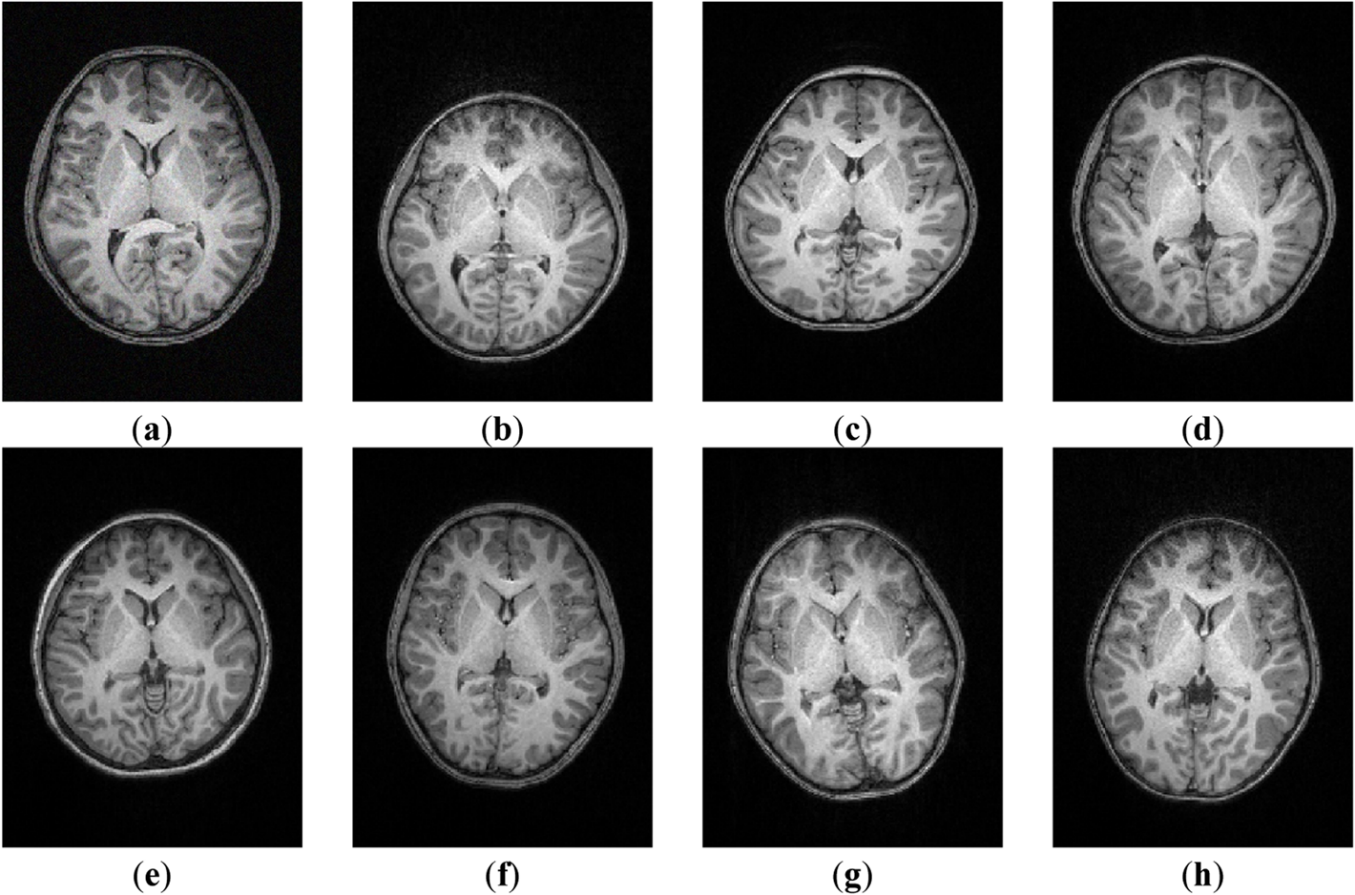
Real human brain MR images
